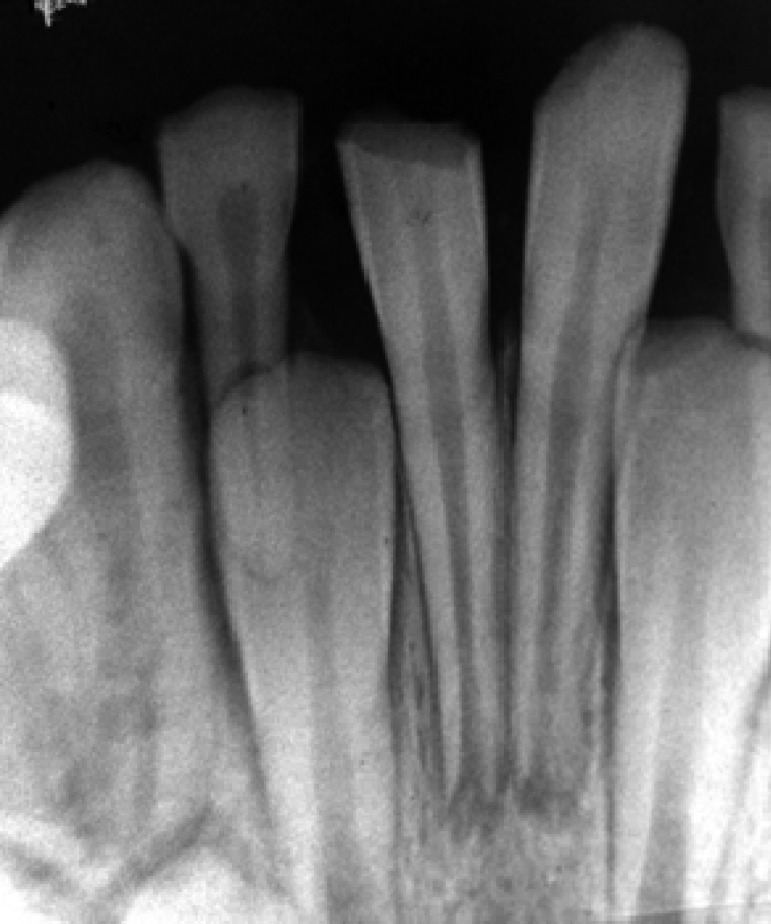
Radiographic image of right and left mandibular incisors after trauma
